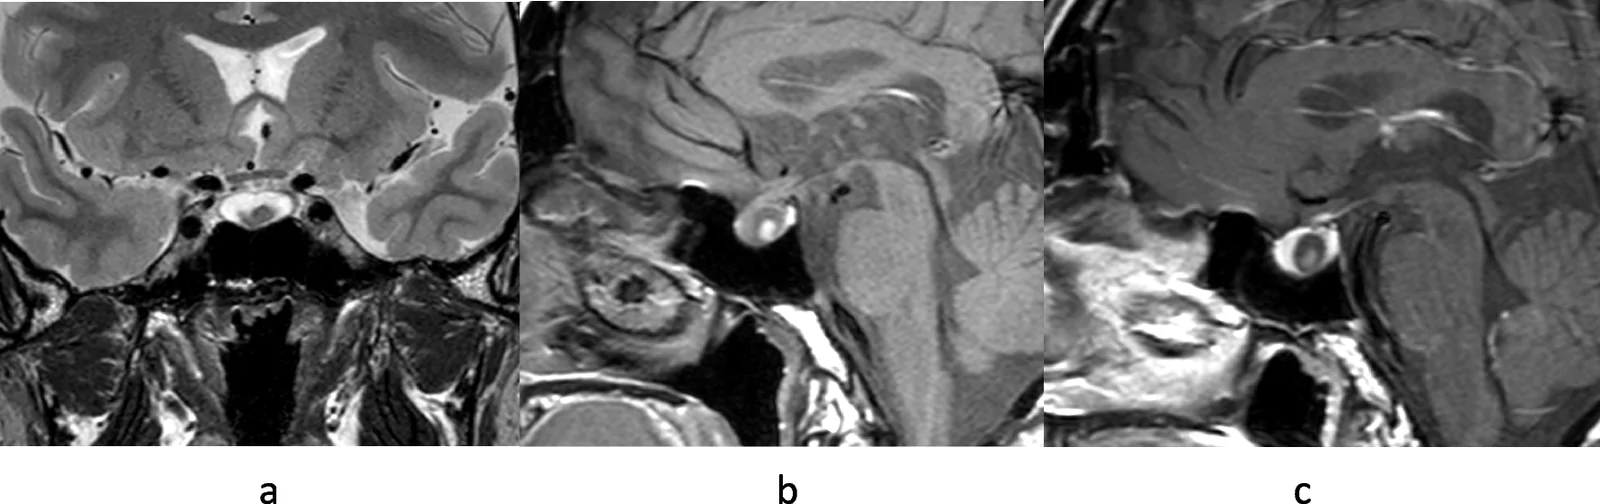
The case of a 43-year-old male with a surgically confirmed RCC with an intracystic nodule. Magnetic resonance images demonstrate an RCC with a nodule in the the cyst that displays low signal intensity on the coronal T2-weighted image (a) and high signal intensity on the sagittal T1-weighted image (b). The cyst and intracystic nodule show no enhancement on the sagittal contrast-enhanced T1-weighted image (c)
